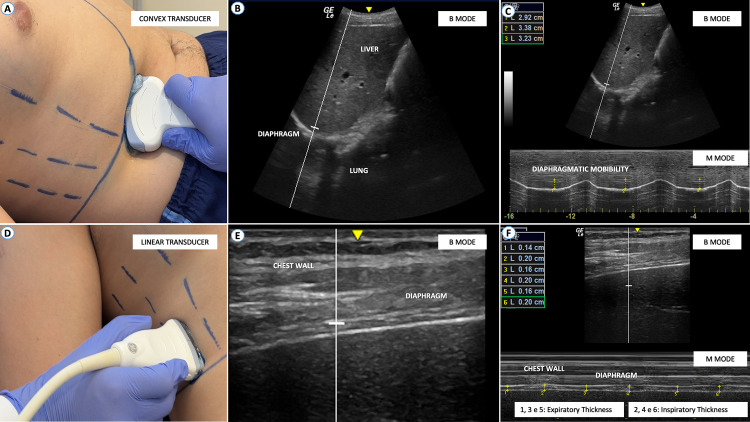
Technique for ultrasound evaluation of diaphragmatic muscle thickness: A) Convex transducer. B) Cake-shaped image on the screen in “brightness – B” mode. C) Mobility assessment in “motion – M” mode. Convex transducers are primarily used for abdominal exams due to their wider and deeper view. D) Linear transducer. E) Cake-shaped image on the screen in “brightness – B” mode. F) Mobility assessment in “motion – M” mode.
